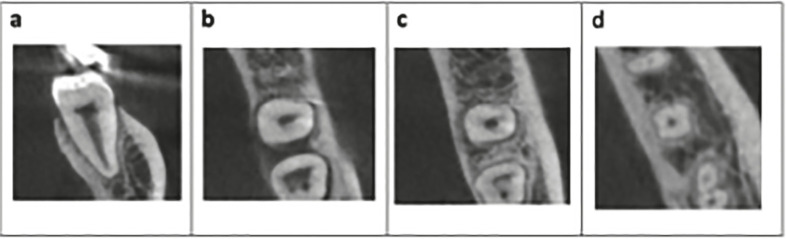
Example of CBCT image of a molar with a single canal. (a) Sagittal section; (b) Coronal, (c) Middle, and (d) Apical third.
